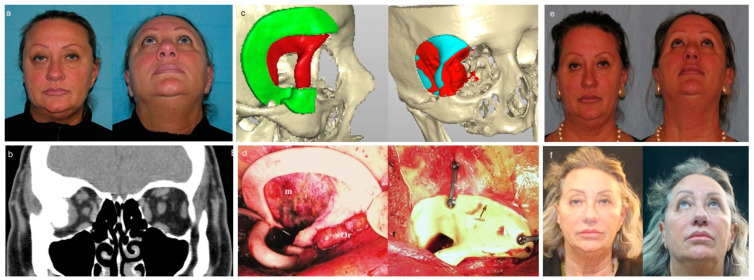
Resection and primary reconstruction of intraosseous meningioma. (a) Preoperative facial views of right exophthalmos and dystopia. (b) Preoperative coronal computed tomogram displays hyperostotic bone owing to intraosseous meningioma. (c) Three-dimensional model based on data from computed tomogram (green, cutting guide; red, custom-made implant, red and light blue, planned resection). (d) Intraoperative use of cutting guide to outline the area of resection that matches the size of the polyetheretherketone implant (m, meningioma; Or, right orbit) and reconstruction of bone defect with a polyetheretherketone patient-specific implant fixed in position with plate and screws. (e) Postoperative facial views show good functional globe position and excellent postoperative cosmetic appearance at 12-month follow-up. (f) Aesthetic outcome at the 36-month follow-up.
